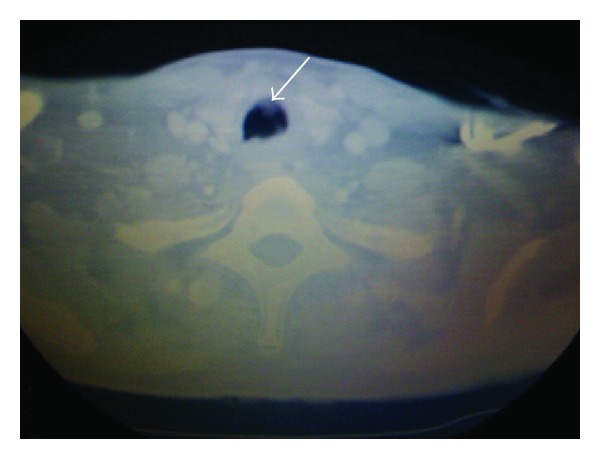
CT scan of the neck showing a haziness in the trachea representing the foreign body.
